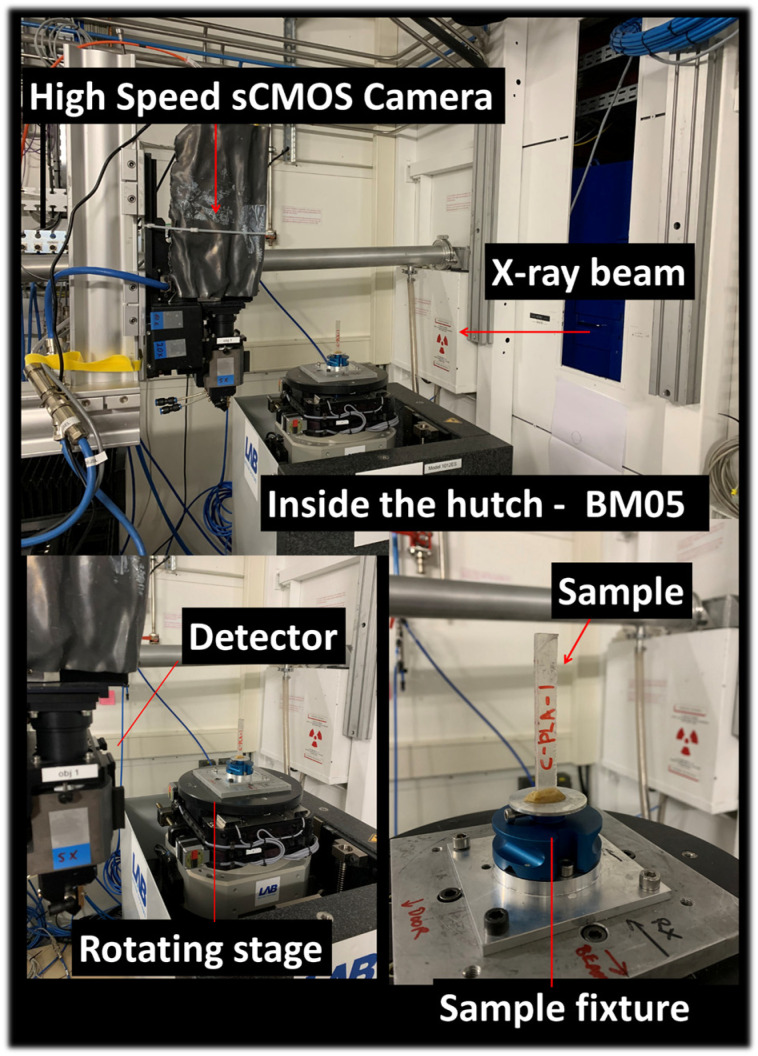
Experimental setup used for synchrotron X-ray microtomography.
